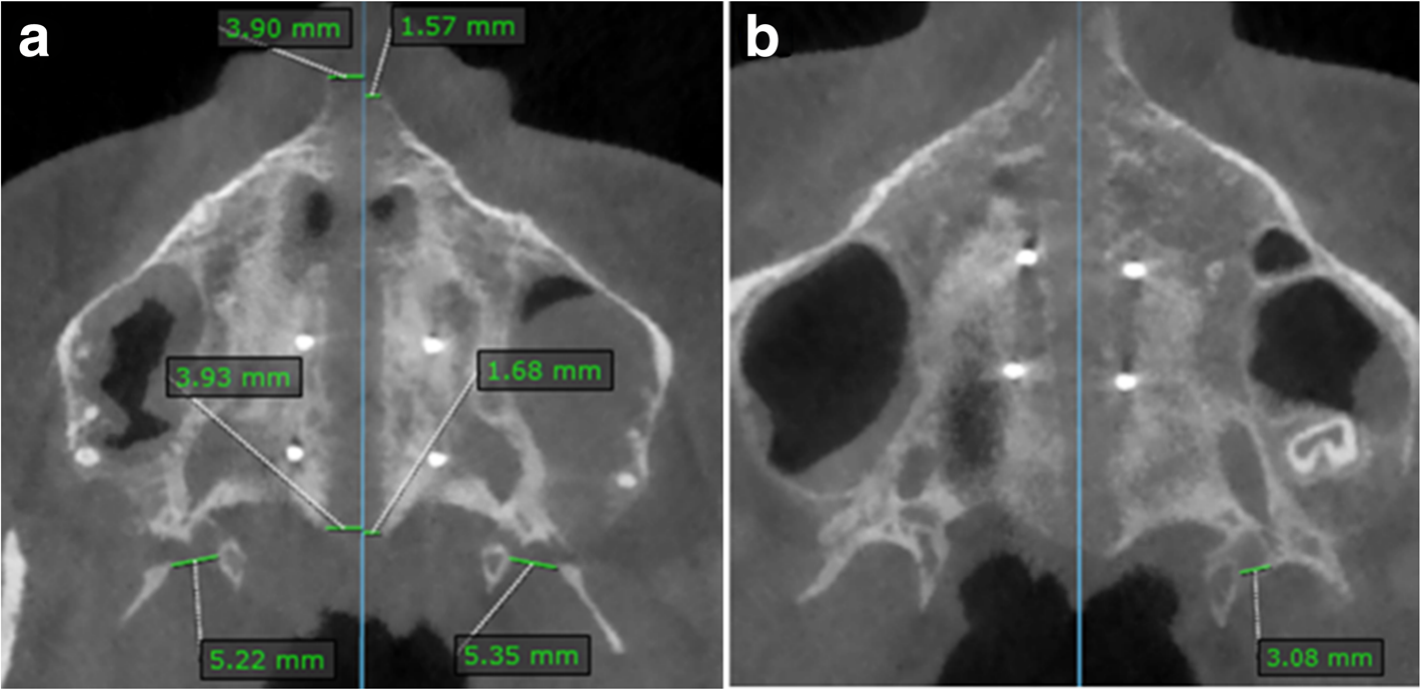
Measurements on axial palatal section on post-expansion CBCT. Blue line, maxillary sagittal plane. a Patient with a complete disengagement of the pyramidal process from the pterygoid plates. b Patient with a partial disengagement of the pyramidal process from the pterygoid plates in the left pterygopalatine suture; the opening is present between the pyramidal process and the medial pterygoid plate
